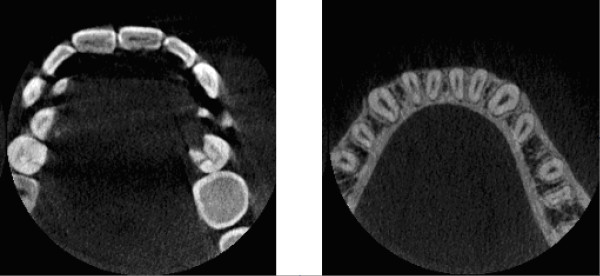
Examples of slices from CBCT volumes.
